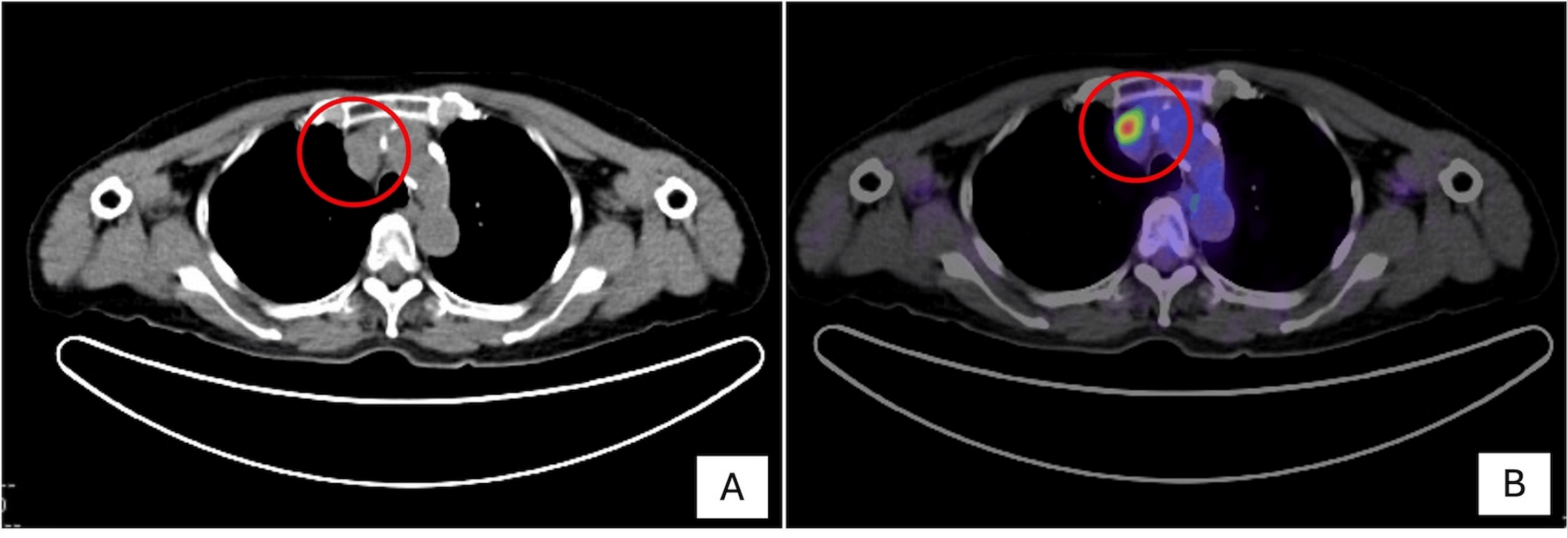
Imaging findings. Axial computed tomography (A) and positron emission tomography-computed tomography (B), displayed according to standard radiological convention, showing intense fluorodeoxyglucose uptake in the anterior mediastinal lesion.
